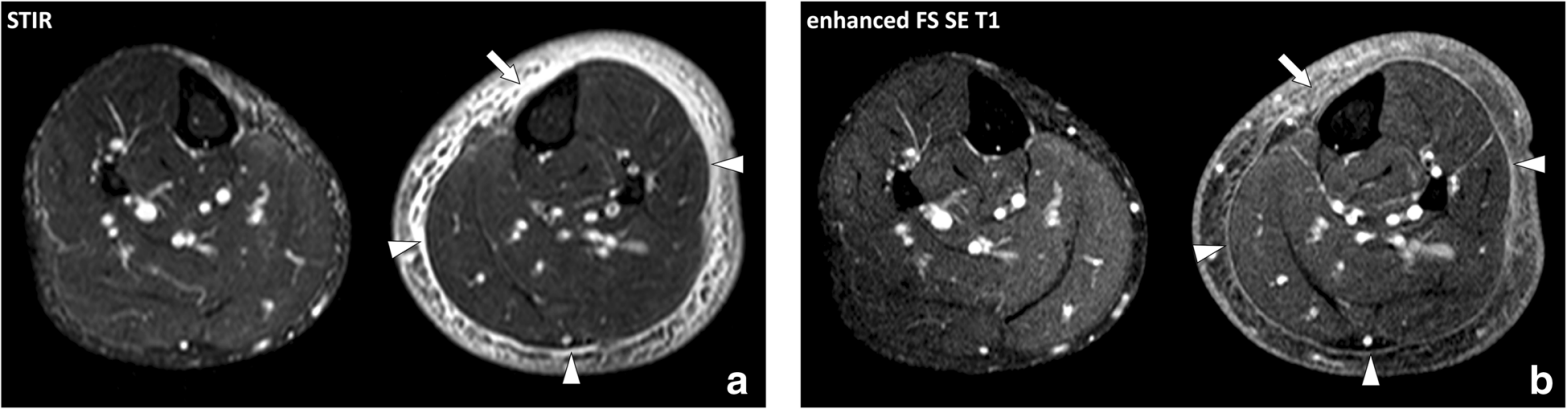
Axial (a) STIR and (b) contrast-enhanced fat-suppressed SE T1w images of the legs of a 55-year-old male with non-complicated cellulitis of the left leg. MRI demonstrates diffuse thickening of the subcutaneous soft tissues with increased fluid content infiltration of the fascia superficialis enhancing after contrast material injection, more severe on the antero-medial part of the leg (arrow). Note the inflammatory infiltration along the interface between the hypodermis and the deep peripheral fascia which conserve normal thickness and signal (arrowheads)
